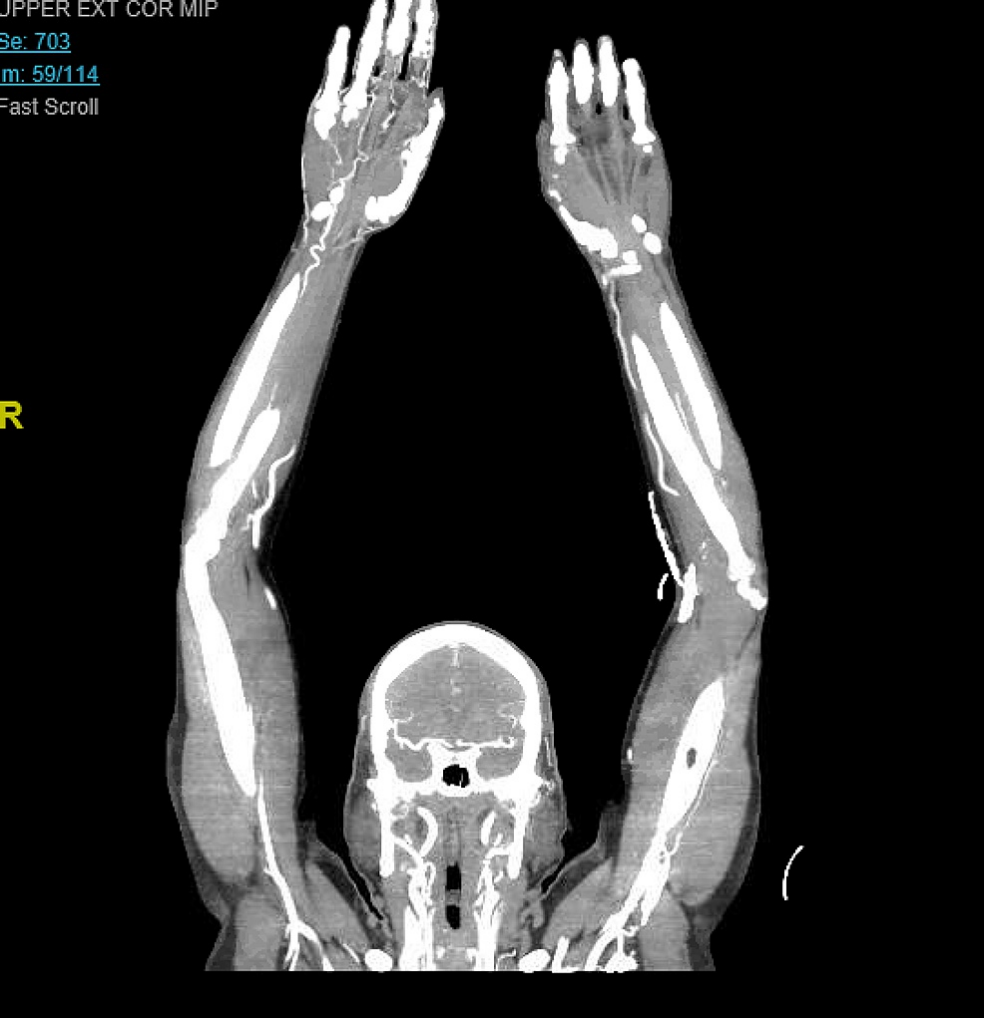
CT angiography of the bilateral upper extremity CT: computed tomography
